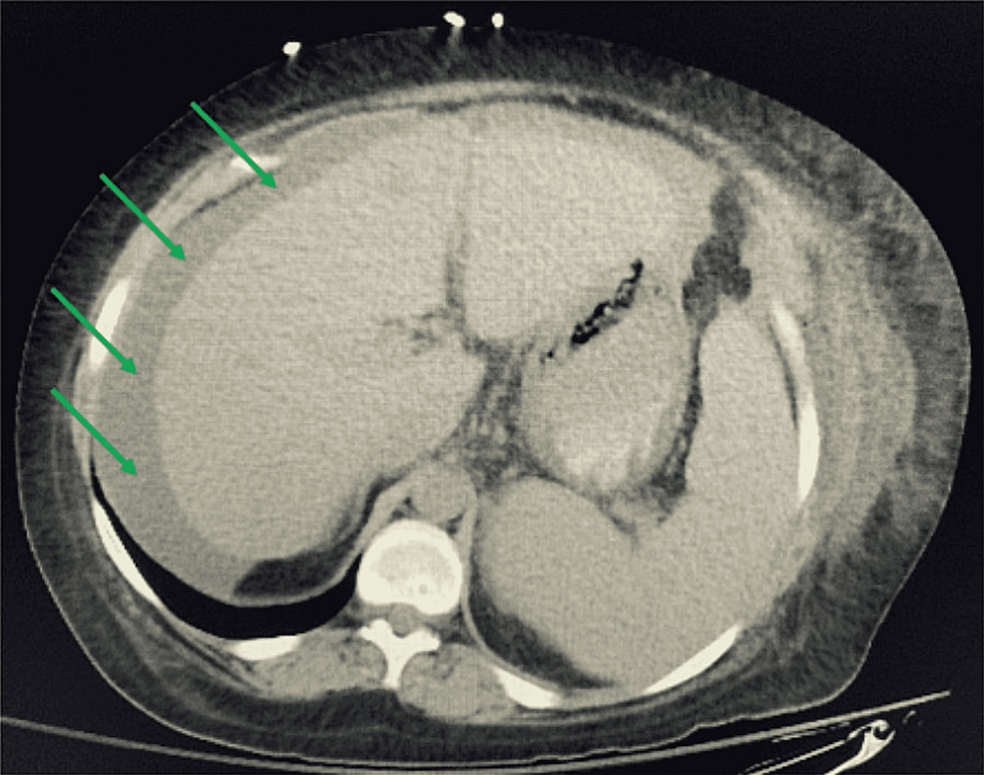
Representative CT image of the abdomen showing ascites. Green arrows indicate the location of ascites.
